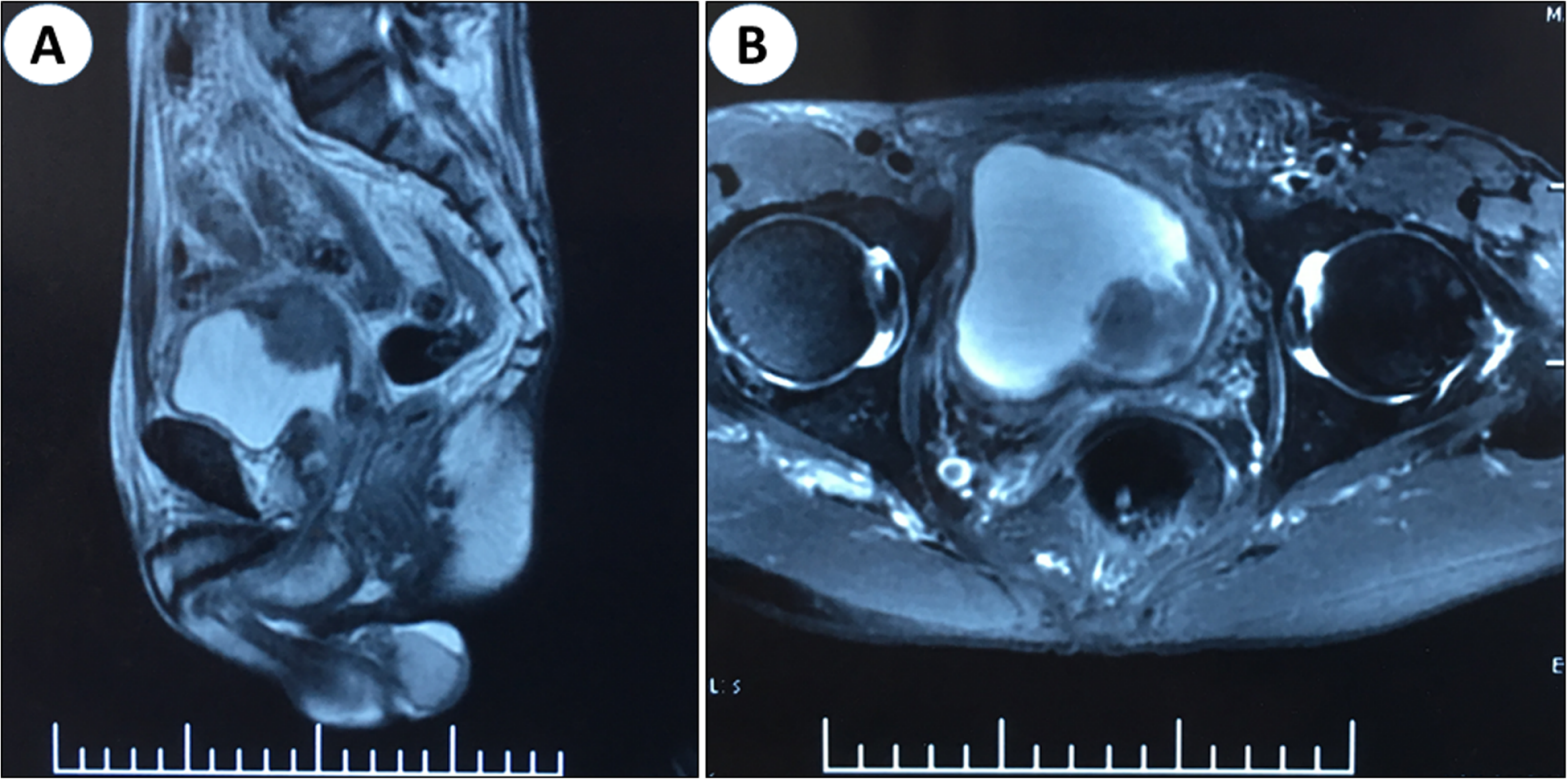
Sagittal (a) and horizontal plane (b) MRI demonstrated an oval-shaped tumor mass invading the entire layer of bladder in the left posterior bladder wall
